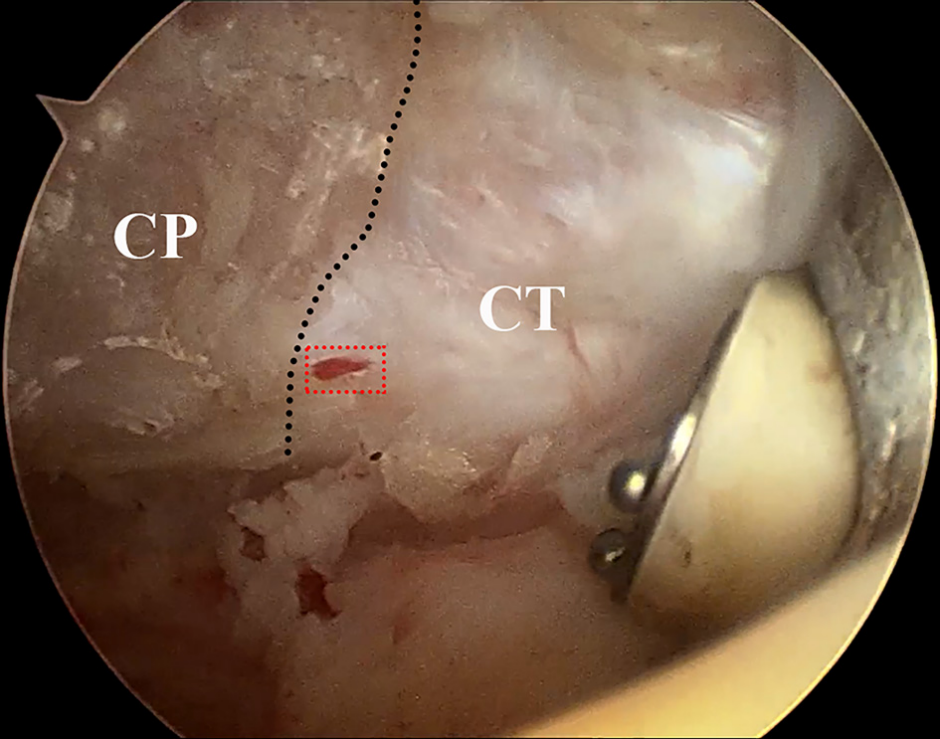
Rich vessels inside the CT near its attachment were observed under arthroscopy
